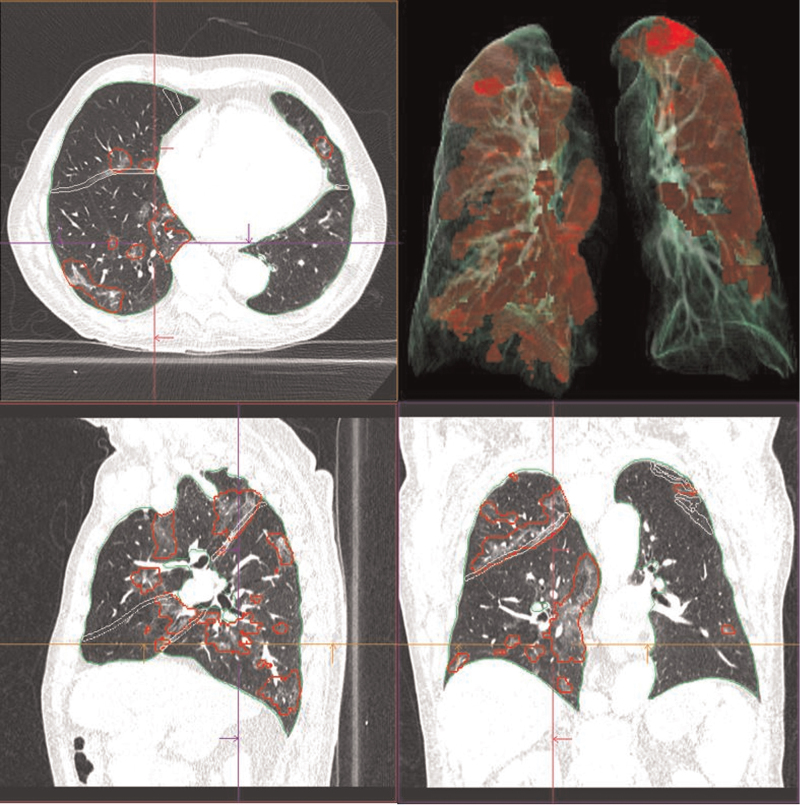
Coronal, axial, and sagittal slices of lungs and 3D reconstruction as an example of visual output of AI-based software tool (*syngo*.via CT Pneumonia Analysis prototype). AI software analysis of a 78-year-old male patient presenting to the emergency ward with fever and increased tiredness. This patient was rt-PCR-positive for SARS-CoV-2.
